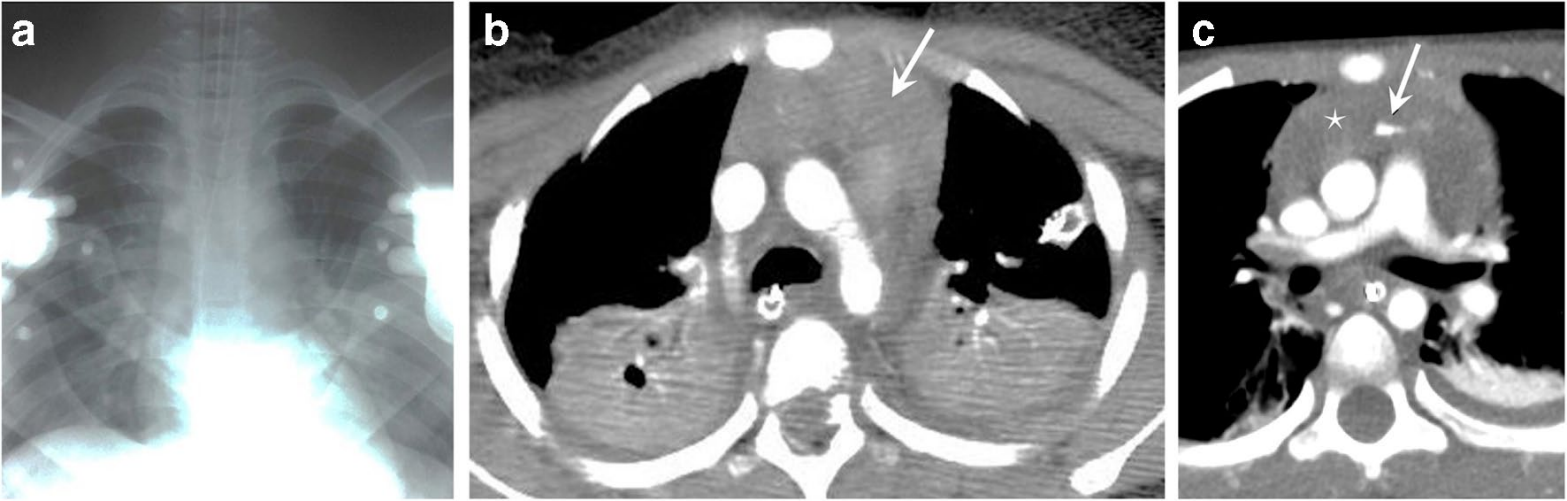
Traumatic non-cardiovascular findings. a Anteroposterior chest radiograph in a 9-year-old boy obtained in the trauma bay demonstrates a mild widening of the upper mediastinum with hazy ill-defined increased paramediastinal opacification. The patient is intubated with a feeding tube in place. External devices are obscuring details. b A 12-year-old girl where an axial contrast-enhanced computed tomography (CT) shows a large mediastinal hematoma (*arrow*) and consolidations/atelectasis in the dependent aspects of both lungs. c A 10-year-old boy with a contrast-enhanced CT showing a large mediastinal hematoma (*asterisk*) with active bleed (*arrow*). Cases courtesy of Dr. David Manson from The Hospital for Sick Children, Toronto, Canada
